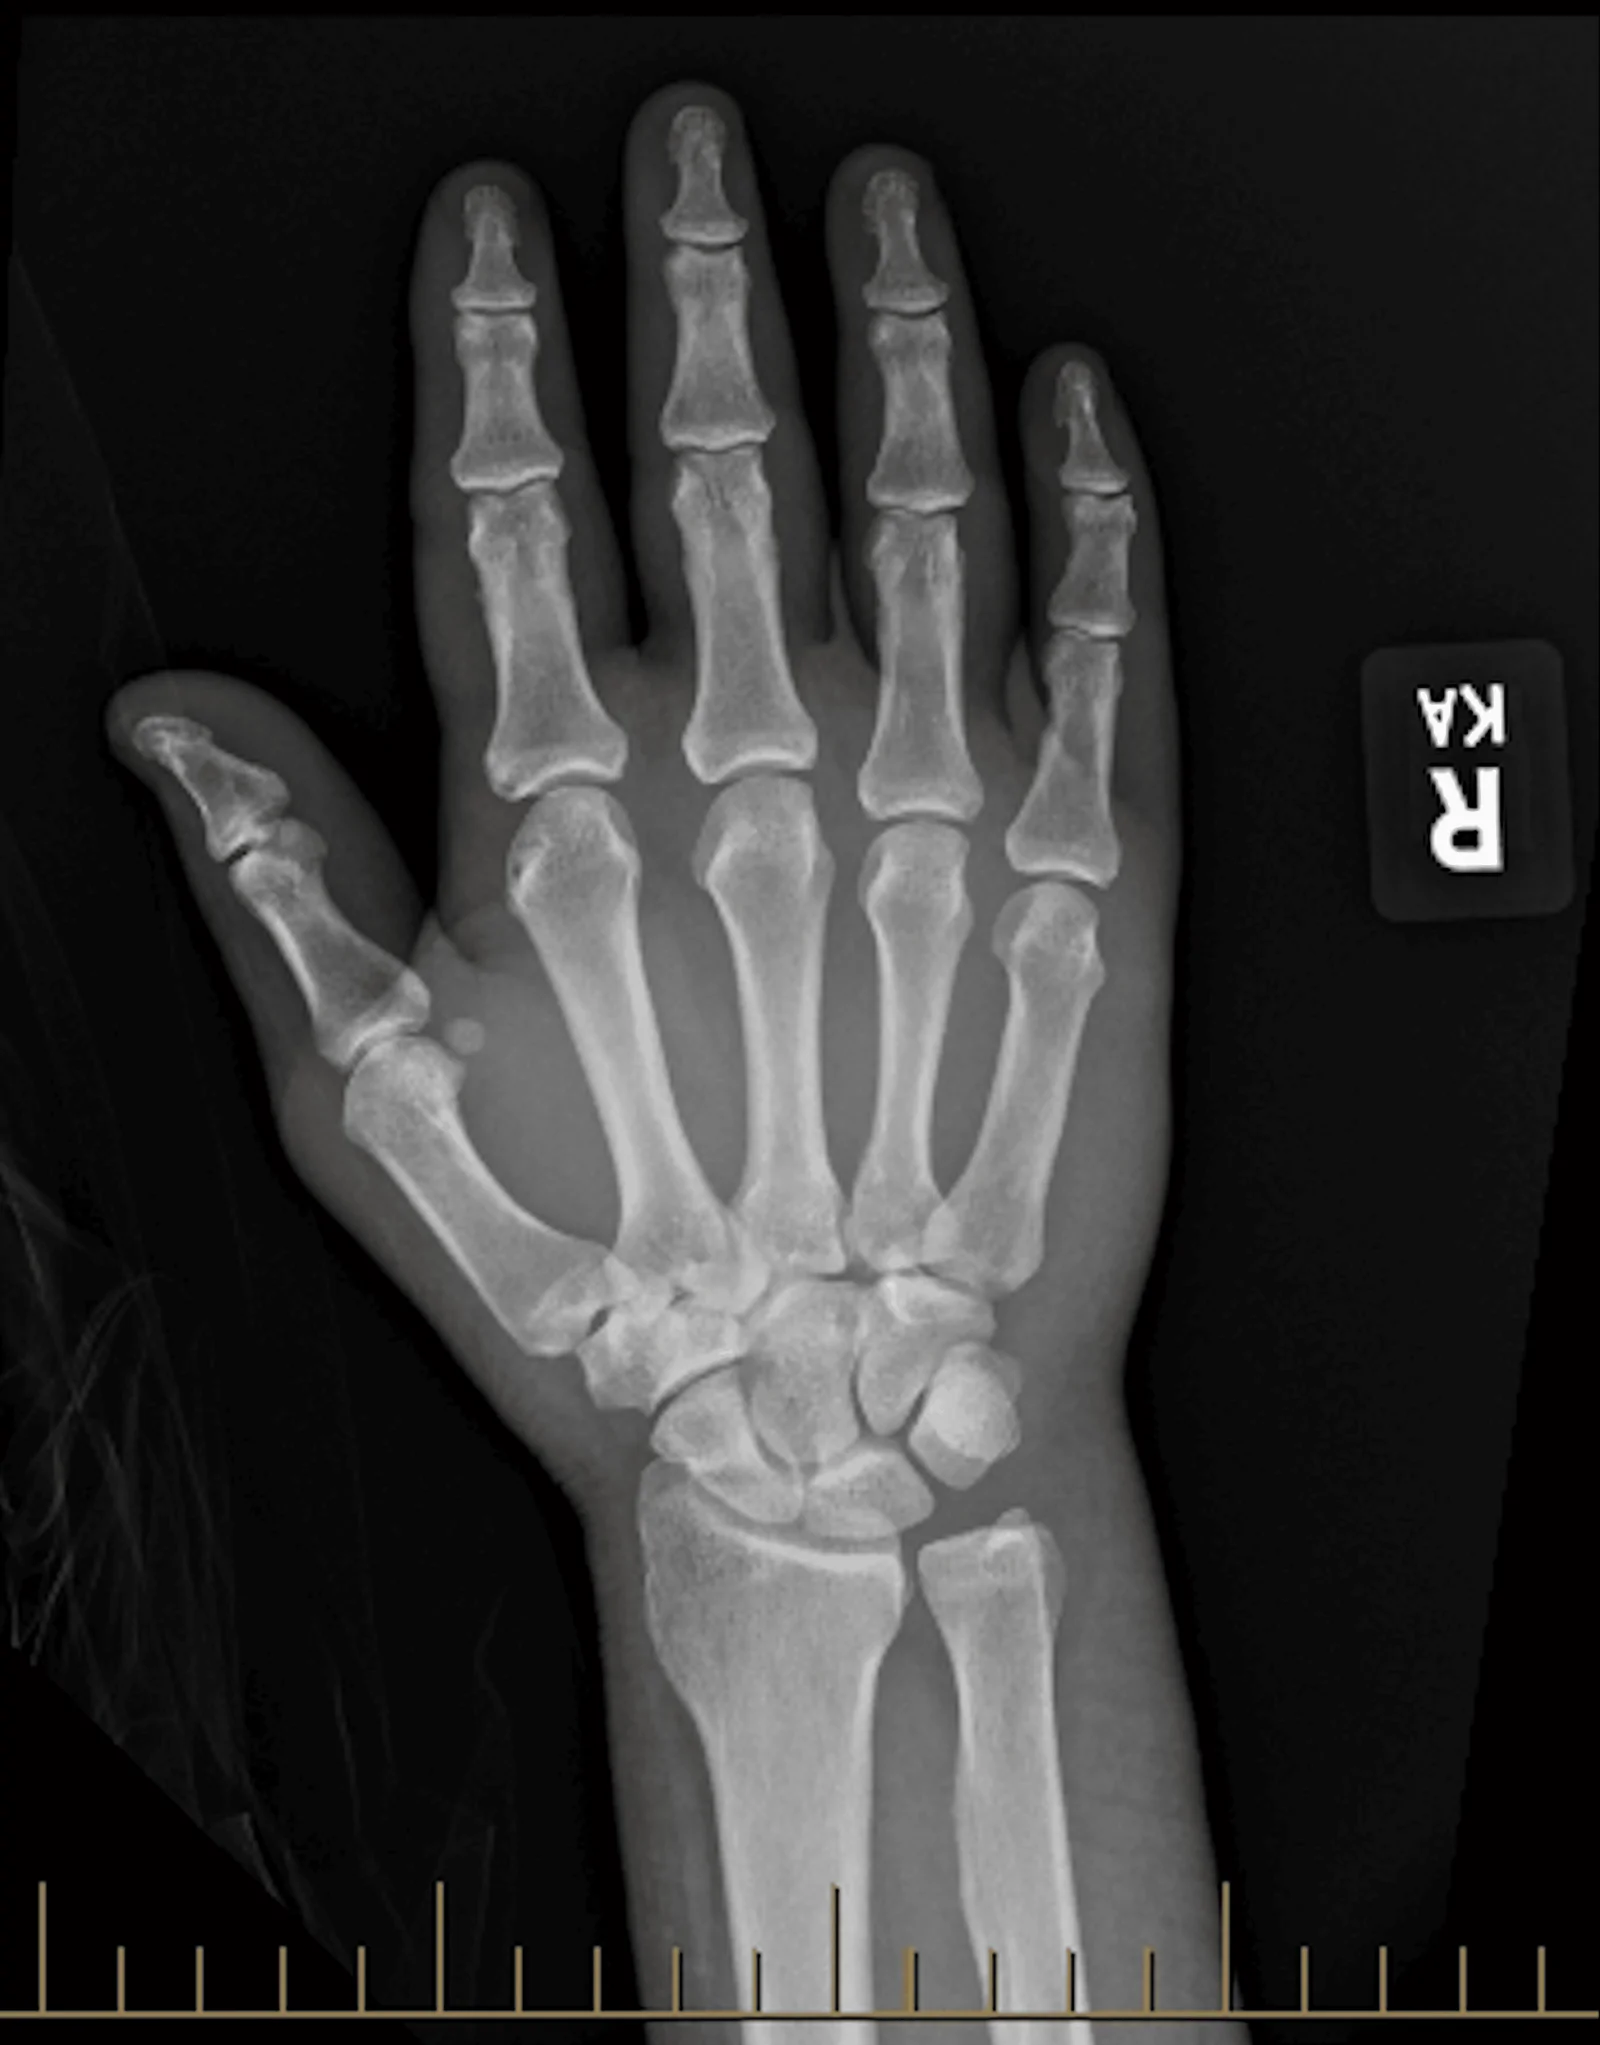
X-ray of the right hand showing no bony involvement on Day 1
